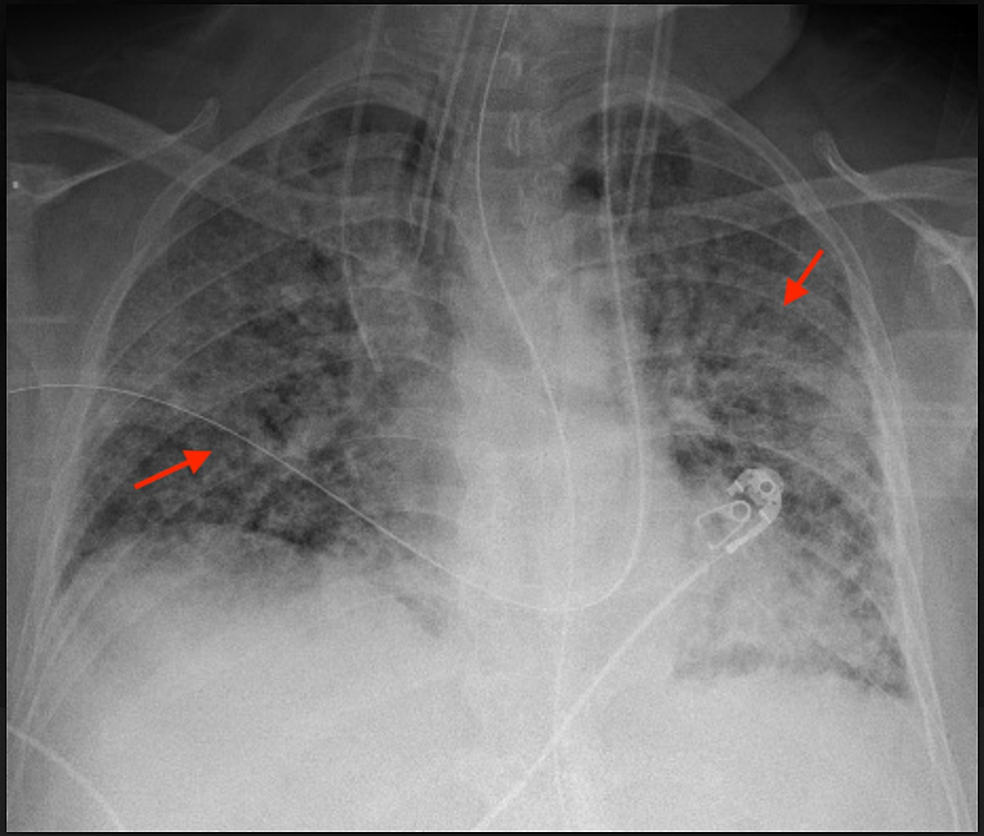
Frontal chest X-ray showing improving diffuse bilateral alveolar infiltrates (red arrows) compared to prior images after initiation of treatment
